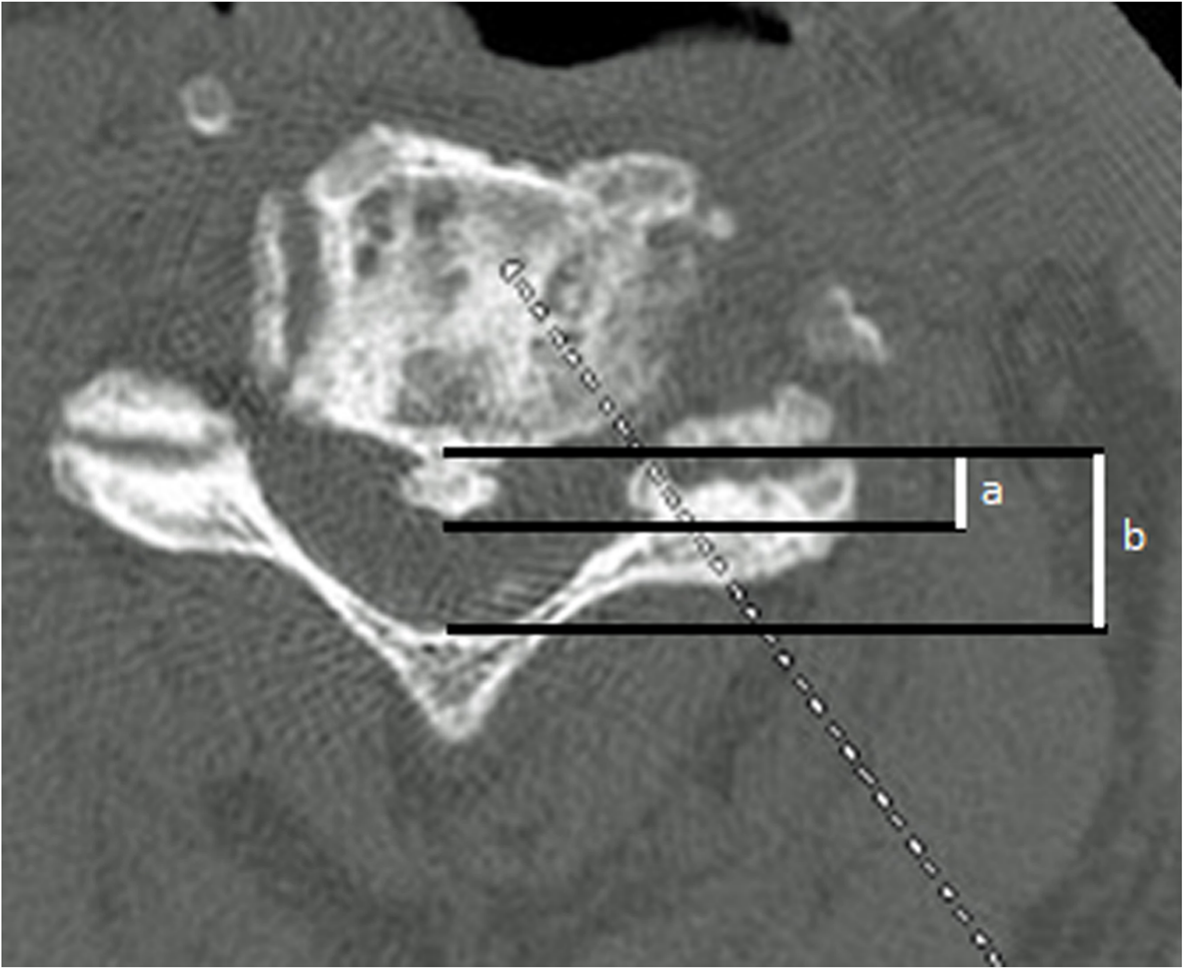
Spinal canal-occupying ratio calculated by dividing maximal ossification thickness (a) by the anteroposterior spinal canal diameter (b) on axial CT imaging
